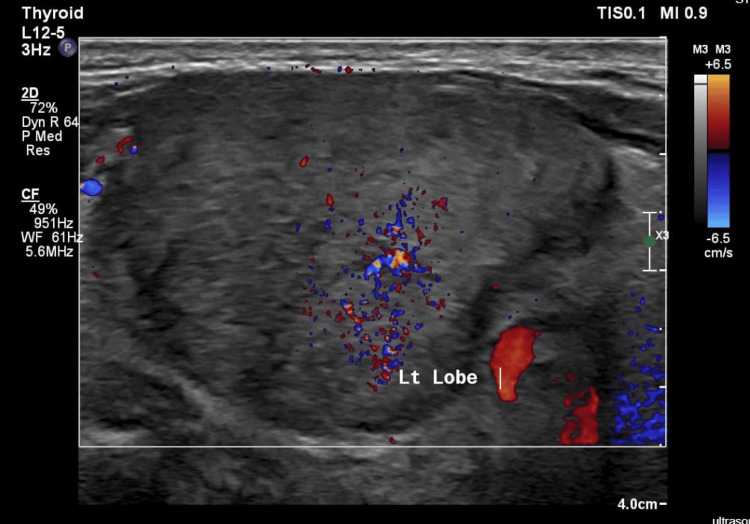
Ultrasound showing the enlarged heterogeneous thyroid with minimal increased vascularity.
